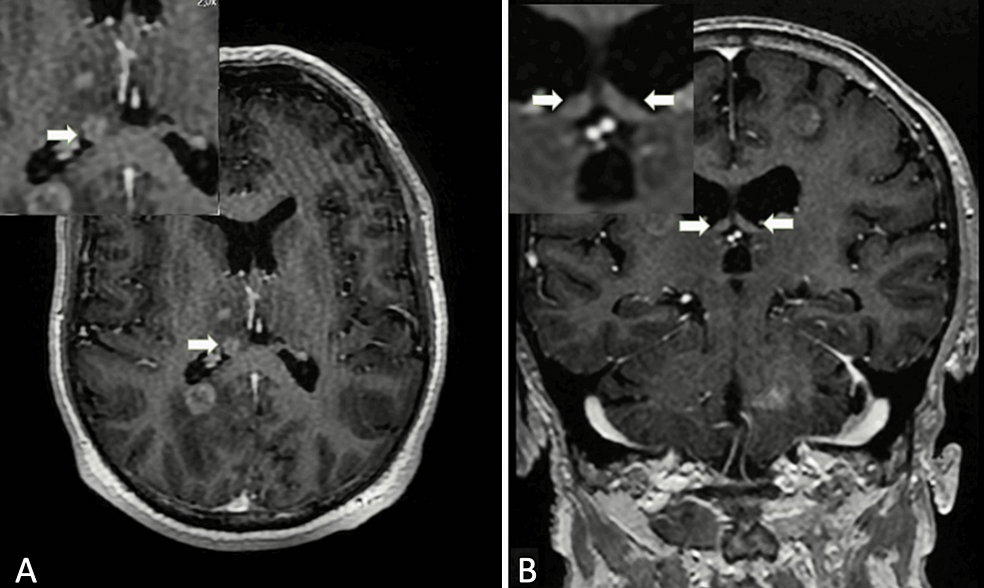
Metastatic lesions (A) Post-contrast axial T1-weighted image showing metastatic lesion localized in the right crus of the fornix (white arrows) in a 60-year-old male with a history of lung adenocarcinoma. (B) Post-contrast coronal T1-weighted image showing two metastatic lesions localized in the bilateral crus of the fornix in a 69-year-old male with a history of small-cell lung cancer (white arrows). 2.0× magnified images of the lesions are shown in the upper left corner.
